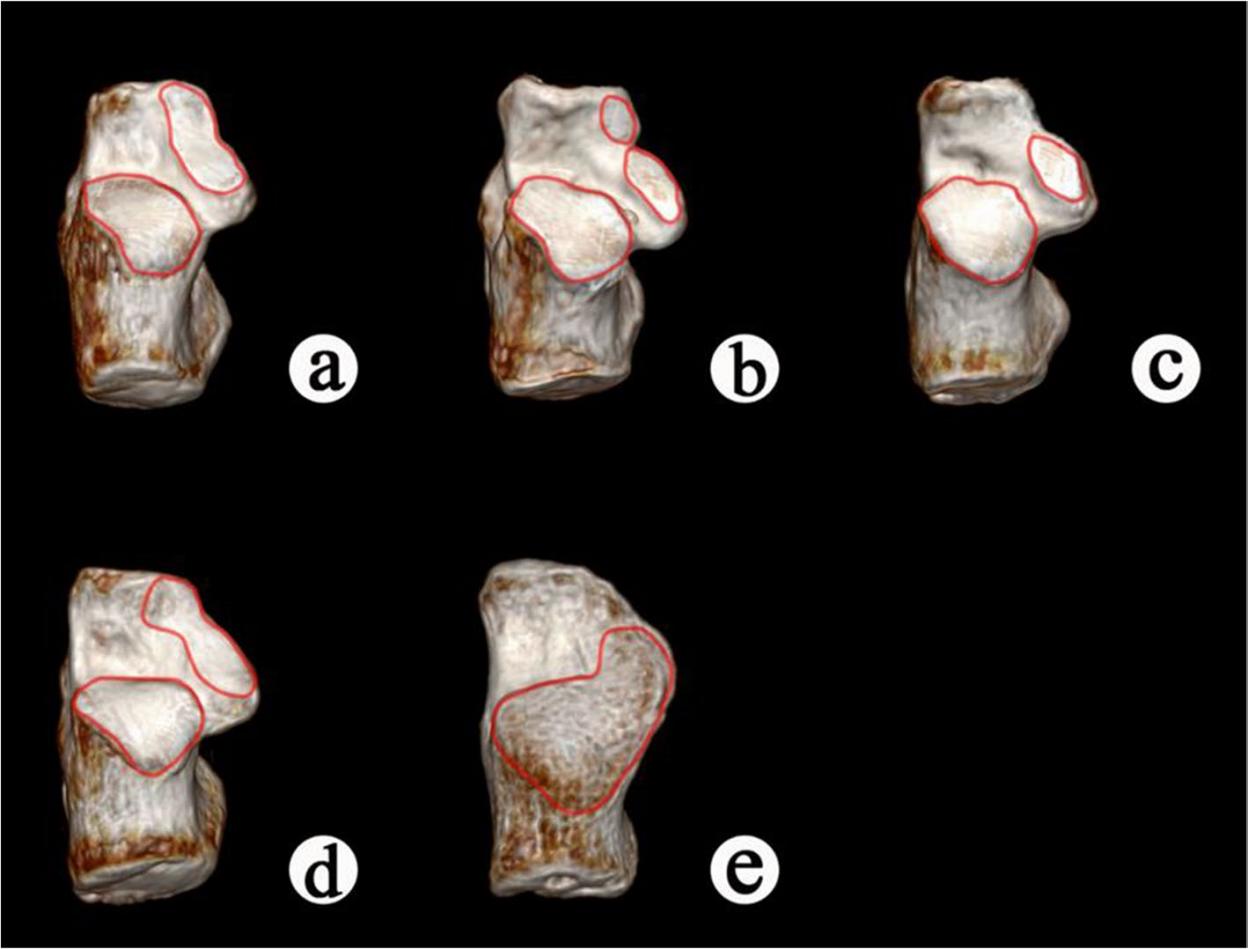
3D reconstruction of subtalar articular surface after CT scanning. a Type I, the anterior and middle facets of calcaneus fused in a continuous and spindle shape facet, and separated from the posterior facet. b Type II, the anterior, middle, and posterior facets of calcaneus were present independently. c Type III, the anterior facet of calcaneus was absent, and the middle and posterior facets were present independently. d Type IV, the anterior and middle facets of calcaneus fused in a continuous and calabash shape facet, and separated from the posterior facets. e Type V, the anterior facet of calcaneus was absent, and the middle and posterior facets were fused in a continuous facet
